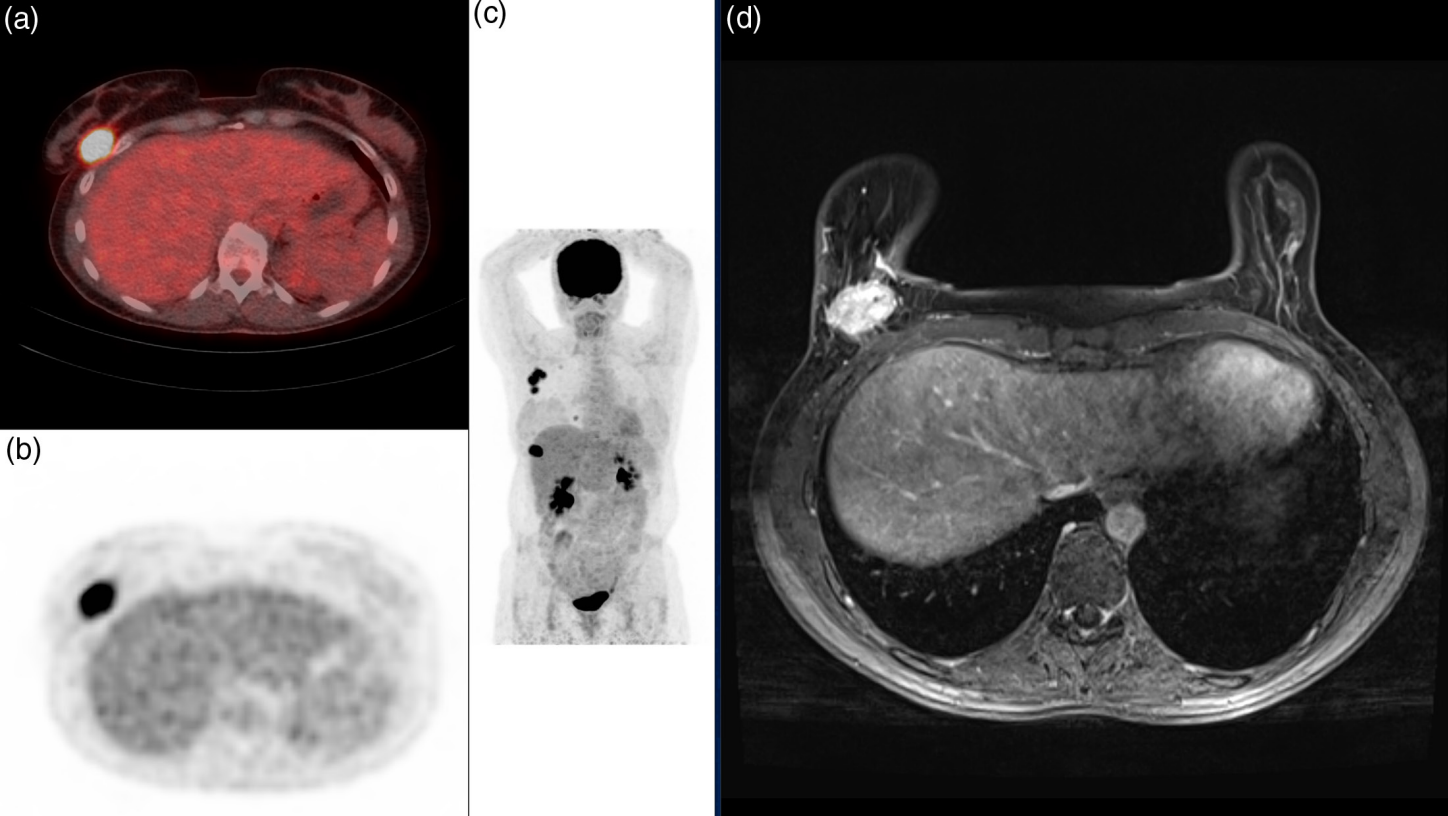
Baseline [^18^F]FDG PET/CT; scan of a 50-year-old patient with right estrogen receptor–positive breast cancer of no special type with a high metabolic uptake (SUV_max_ primary tumour = 9.25). The breast lesion was detected on [^18^F]FDG PET/CT (a, axial fusion image; b, axial PET image; c, maximum intensity projection image) and on MRI (d, axial contrast-enhanced T1-weighted MRI exam). CT, computed tomography; [^18^F]FDG, [^18^F] fluorodeoxyglucose; SUV_max_, maximum standardised uptake value.
